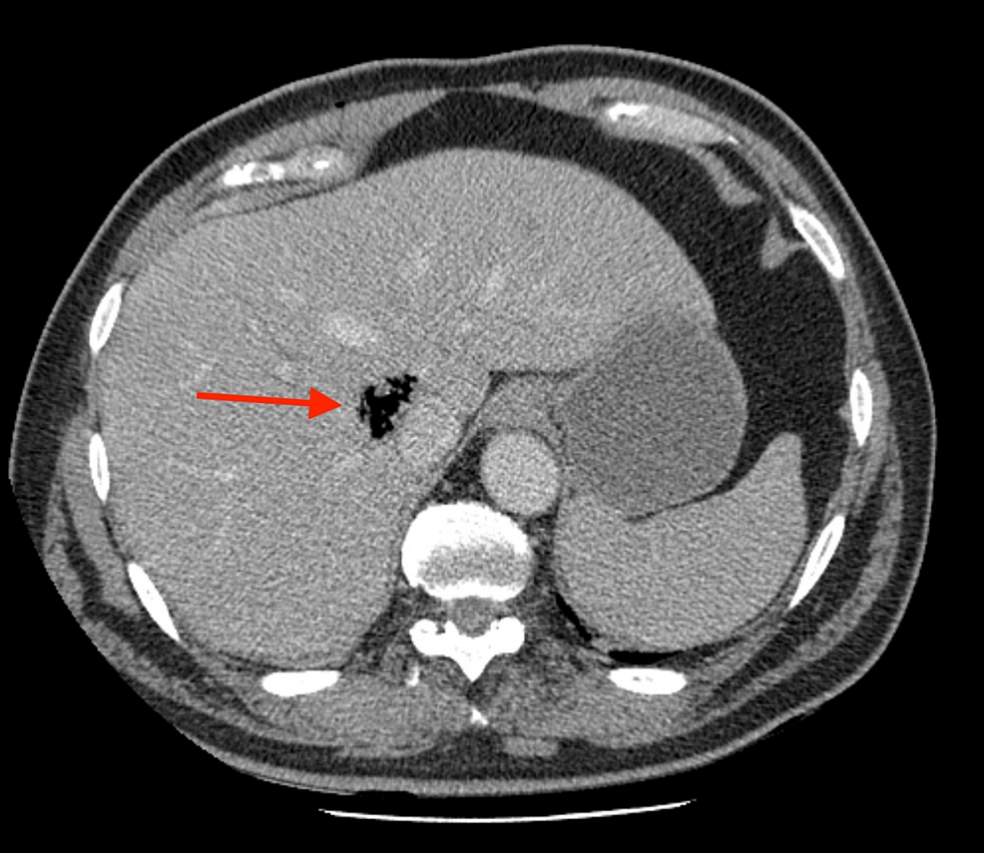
Contrast-enhanced computed tomographic (CT) of the abdomen Gas filled cavity in segment VIII of the liver near the inferior vena cava (red arrow)
